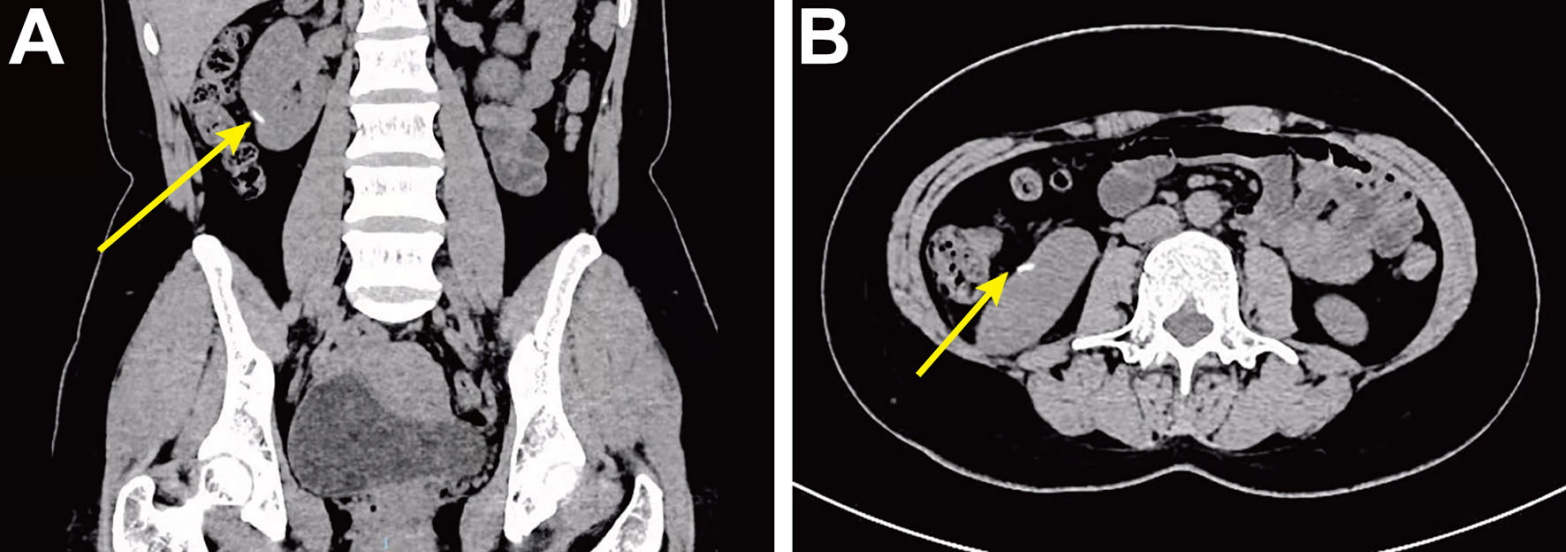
Postoperative follow-up high-density CT shows surgical area marked by hemolok (solid yellow arrow). (A) Coronal CT. (B) Axial CT.
